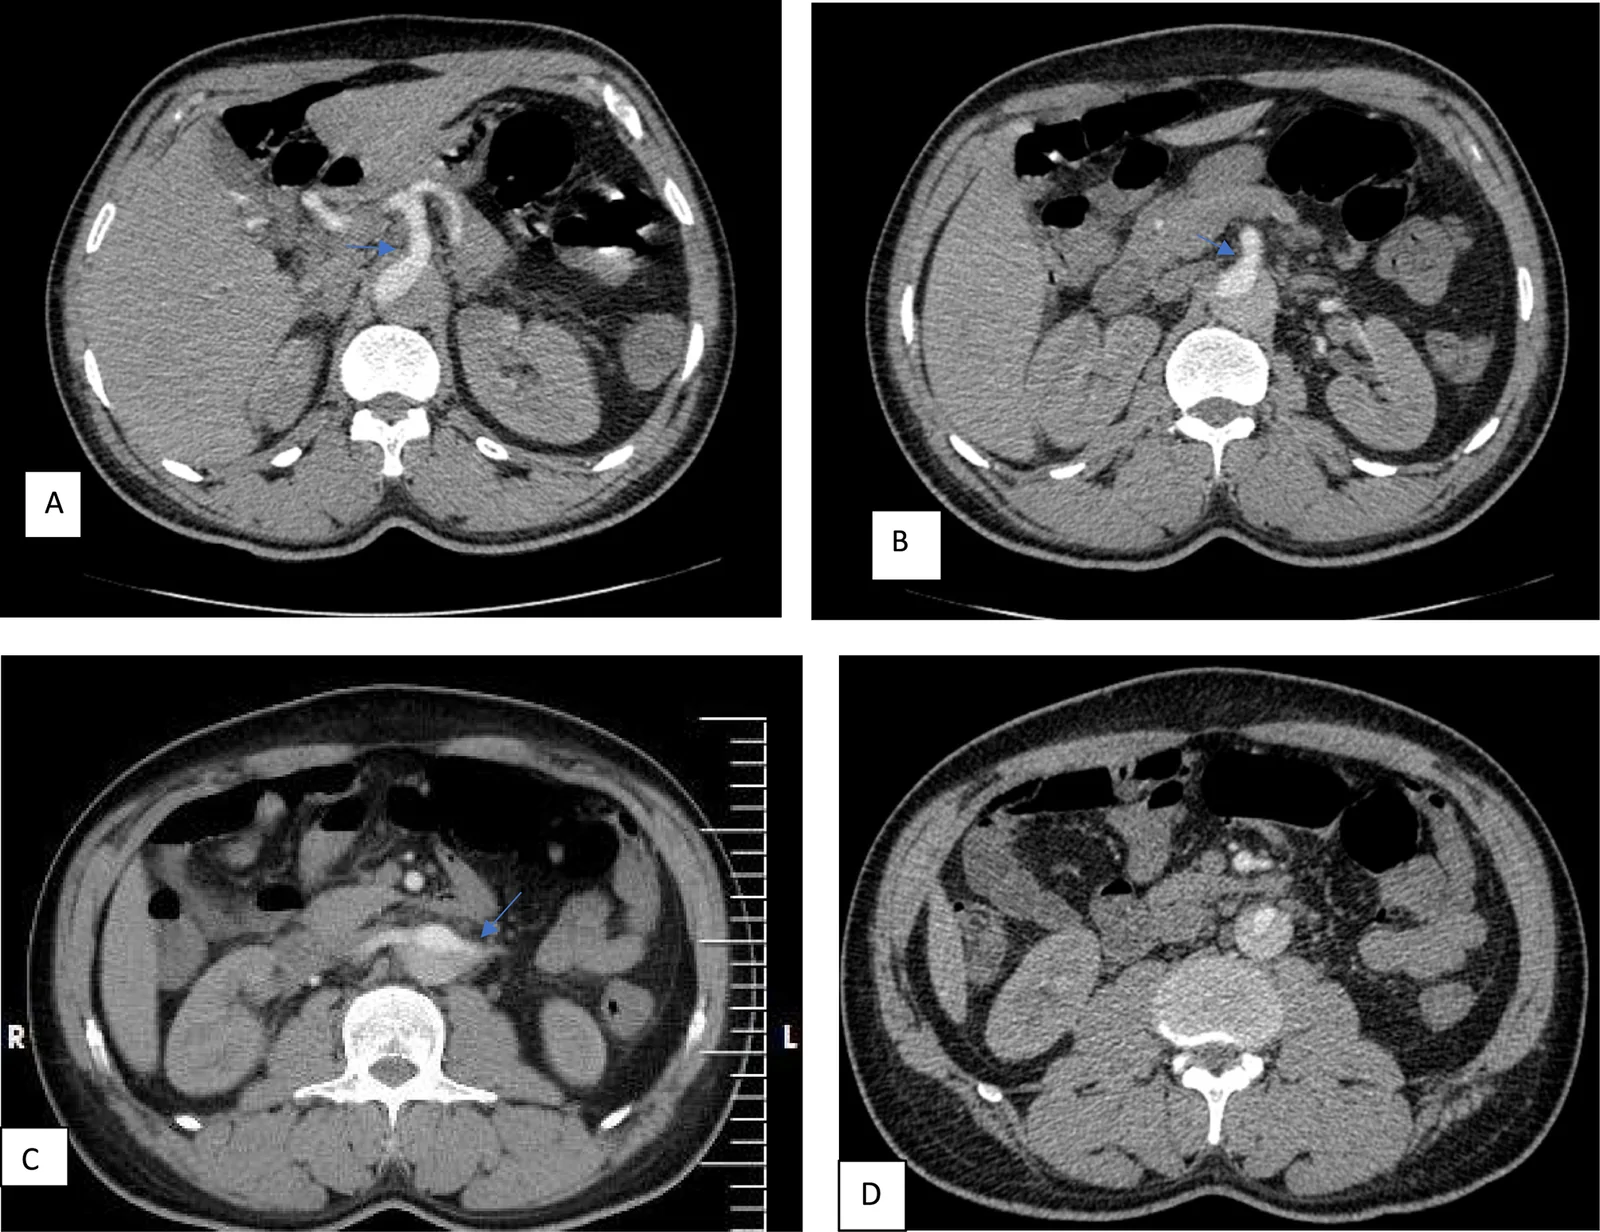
A-D: Serial Post-contrast axial CT (upper abdomen slices) showing the distal extent of the dissection with progressive narrowing of the abdominal aorta. A shows the origin of the coeliac artery (arrow); B, the Superior mesenteric artery (arrow); C, the renal arteries with mural thrombus within the false lumen compressing the true lumen, resulting in narrowing of the left renal artery (arrow); D is the most distal cut of the infrarenal aorta demonstrated
